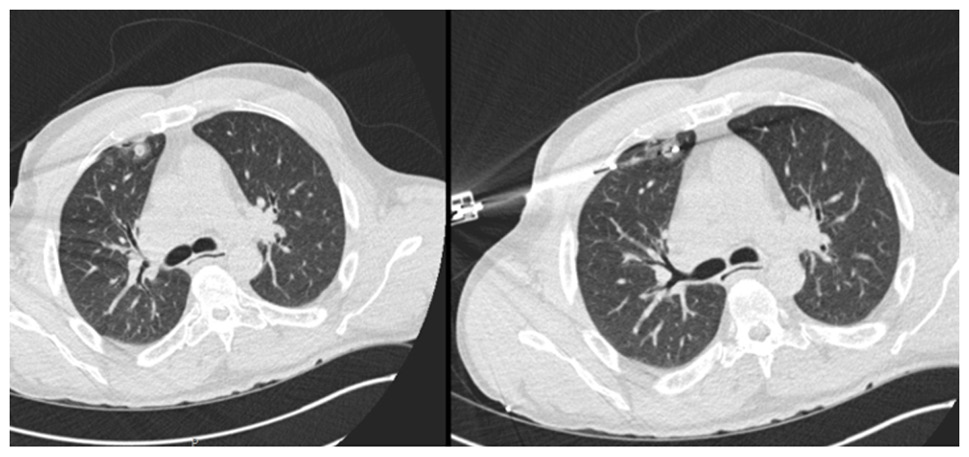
Axial computed tomography scan demonstrating a right upper lobe lung metastasis (left panel), subsequently treated with image-guided ablation (right panel).
